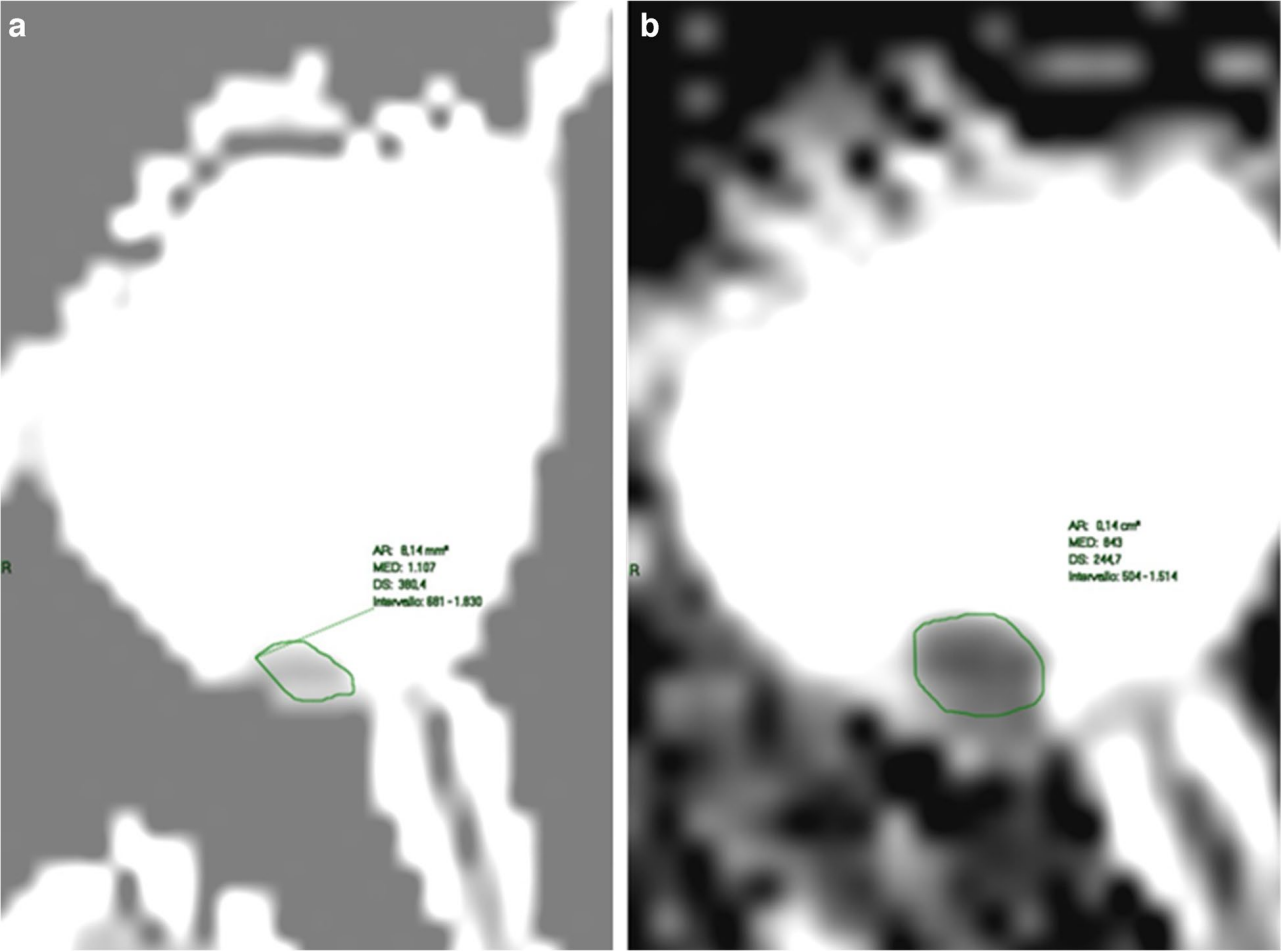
Axial diffusion-weighted MRI of a girl at 19 months (a) and 30 months (b), previously diagnosed with retinocytoma. The first scan (a) demonstrates a high ADC value, whereas the subsequent scan (b) shows a reduced ADC value, reflecting changes in the tumor over time. *MRI*, magnetic resonance imaging
